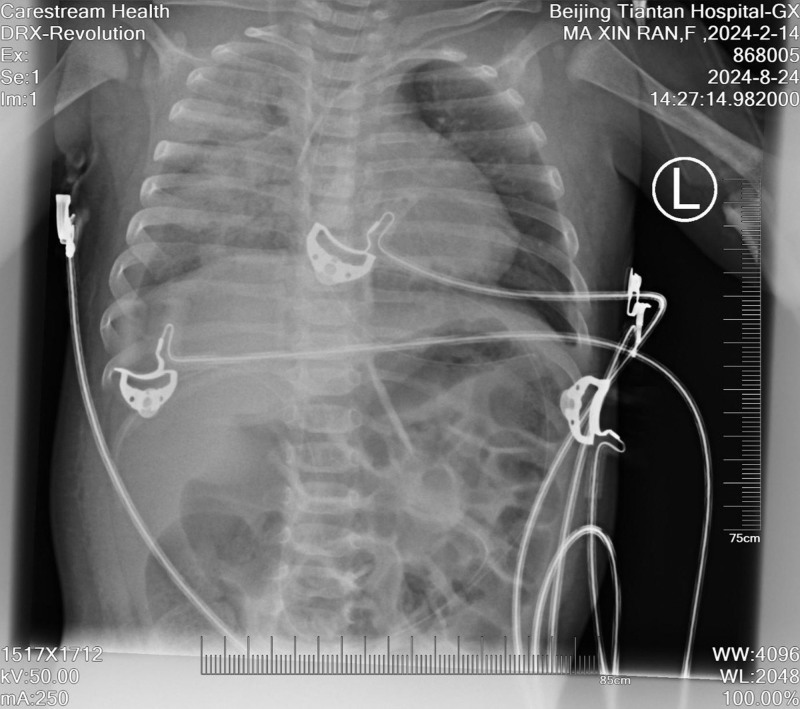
Postoperative chest X-ray image indicating pleural effusion and pulmonary edema in a 6-year-old patient after endoscopic third ventriculostomy. The image shows coarse lung markings and fluid accumulation.
